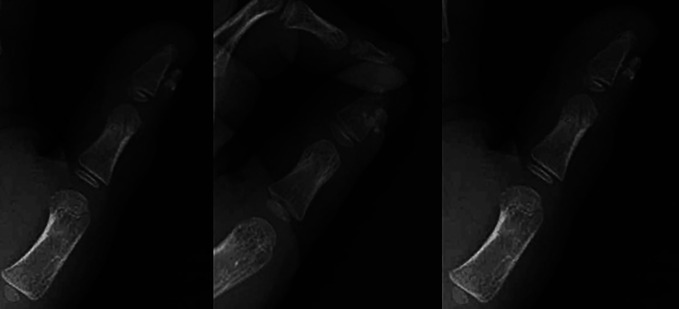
Radiographs showing three views of the left hand demonstrating dorsal calcification overlying the distal phalanx of the thumb.
